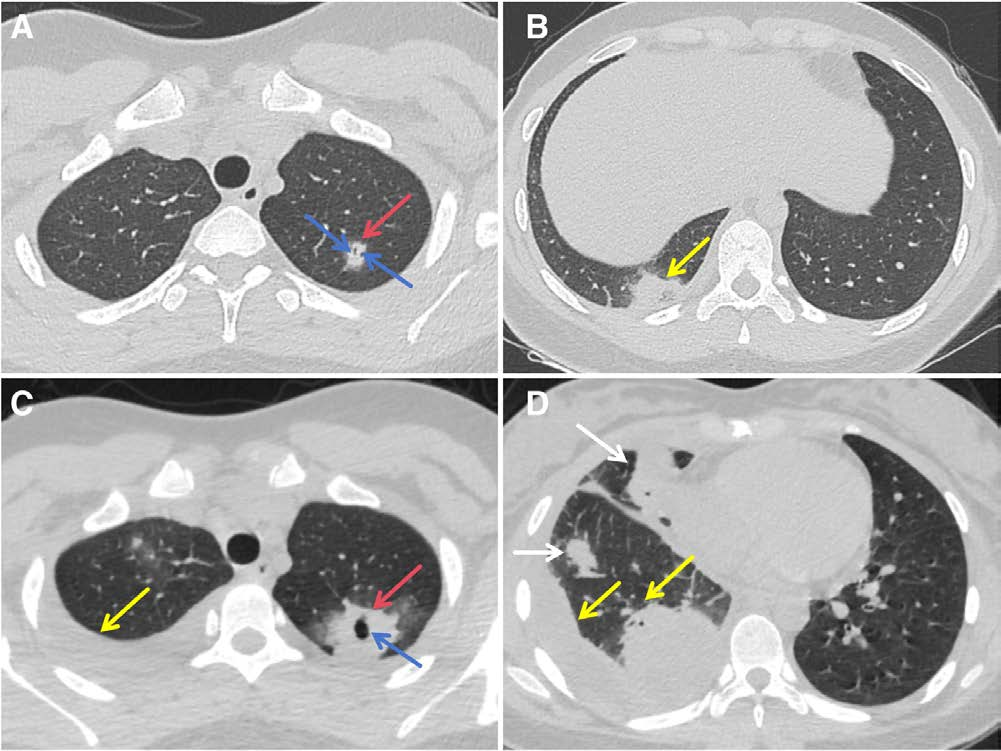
Changes in chest CT findings of the patient before a definite diagnosis of Lemierre syndrome. (A and B) The chest CT findings on May 25, 2024 revealed a nodule (A, red arrow) with 2 small cavities (A, blue arrows) in the left upper lung, and a nodule (B, yellow arrow) in the right lower lung, with no significant abnormalities in the remaining lungs. (C and D) After admission, the disease progressed rapidly, and the chest CT findings on May 29, 2024 revealed that the nodule in the left upper lung was enlarged compared with the previous one (C, red arrow) and the cavity was enlarged (C, blue arrow), and the pleural effusion appeared in the right upper lung (C, yellow arrow). Solid lesions (D, white arrows) and encapsulated pleural effusions (D, yellow arrows) occurred in the right lower lung. CT = computed tomography.
